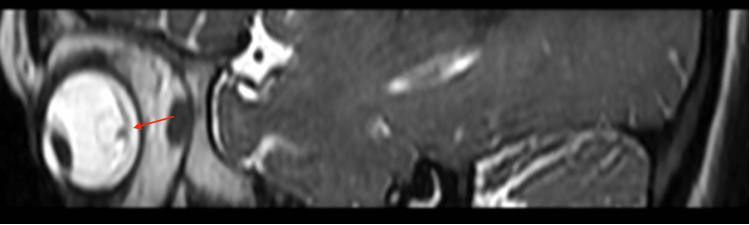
MRI of the lateral view of the eye showing cyst in the right eye
